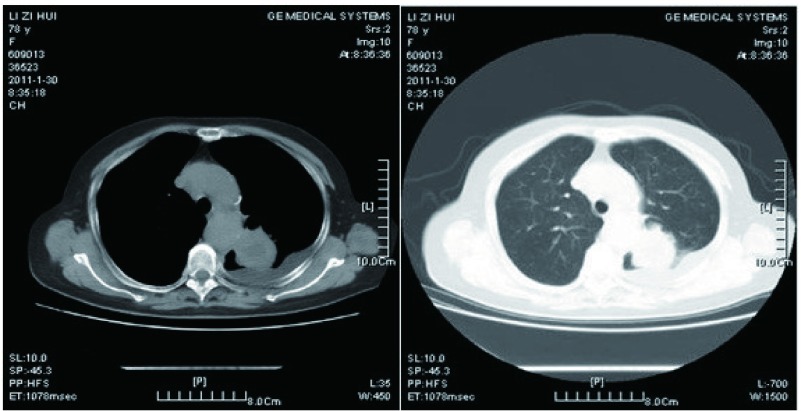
胸部CT示：左上肺肿块，左侧胸腔积液 The chest CT scan revealed a mass on the left upper lobule of the lung with left pleural effusion
